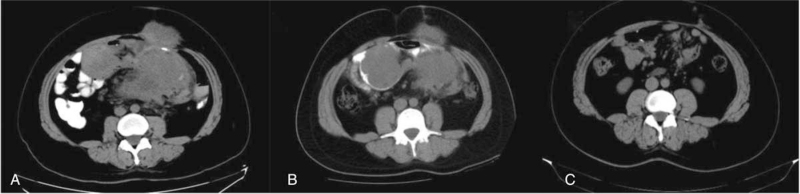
(A) The abdominal enhanced CT scan (February 2020) showed a soft tissue mass in the abdominal wall and an irregular mesenteric soft tissue mass with internal fistula. (B) The abdominal enhanced CT scan (August 2020) showed intraperitoneal abscess was smaller than before. The abdominal wall soft tissue mass was the same as before. There were multiple irregular nodules in the mesentery and multiple small lymph nodes behind the peritoneum. (C) The abdominal enhanced CT scan (July 2021) showed there were abdominal wall scars and high-density staples, and the anastomotic wall was slightly thickened. CT = chest computed tomography.
